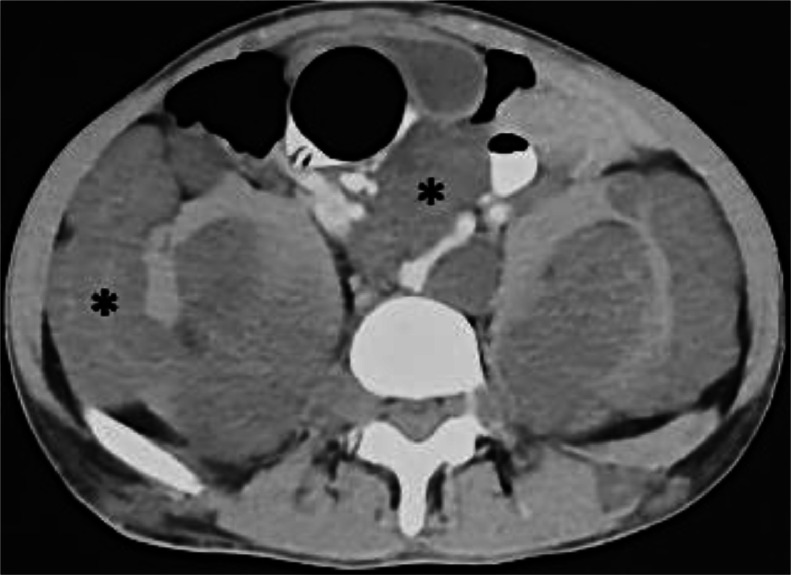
Axial section of the abdominal CT scan revealed multiple masses with low density compared with muscle at intraperitoneal and extraperitoneal (asterisk).
